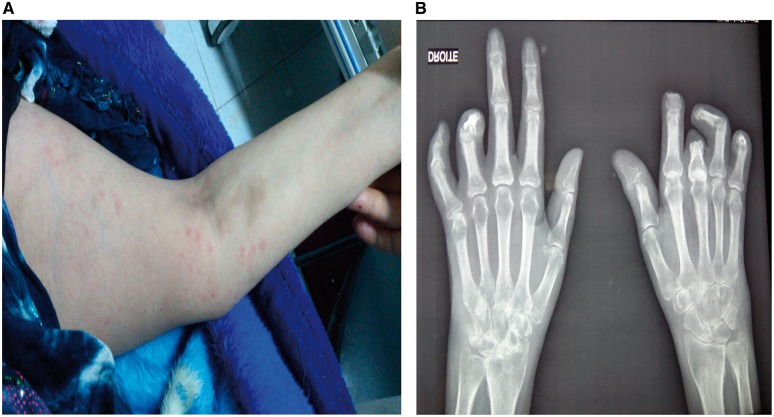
Destruction of the distal IP joints with ankylosis of the right wrist and evanescent rash. (A) Erythematous macules involving the right arm. (B) A radiograph of the hands shows severe structural destruction in the distal IP joints of both hands with erosions and ankylosis of the right wrist.
